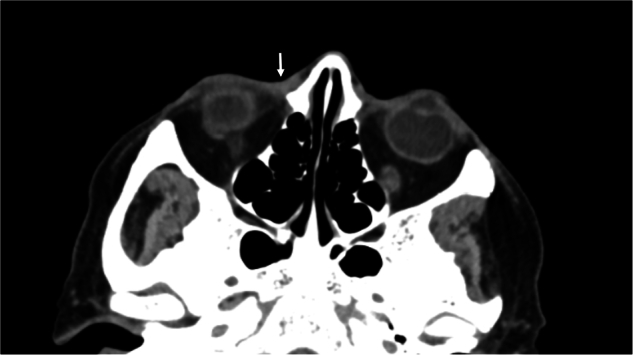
Axial CT image of patient 3. Demonstrating pre-operative right anterior skin thickening with anterior orbital extension (arrow).
